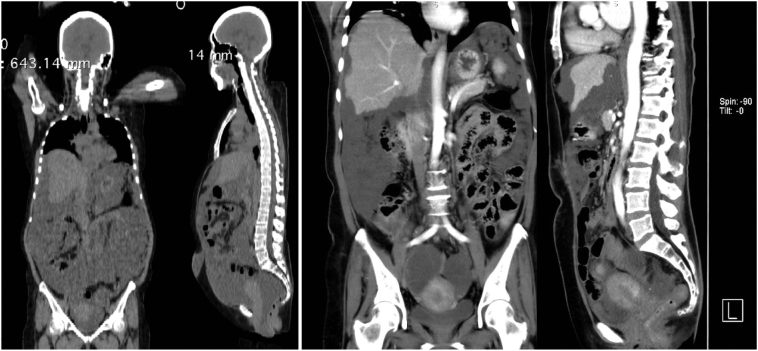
CT-Scan whole abdomen: A. Pre surgery (July 2020) showing ascites in the abdominopelvic cavity, B. post surgery (October 2020) showing fluid accumulation and multilocular cystic mass in the abdominopelvic cavity.
